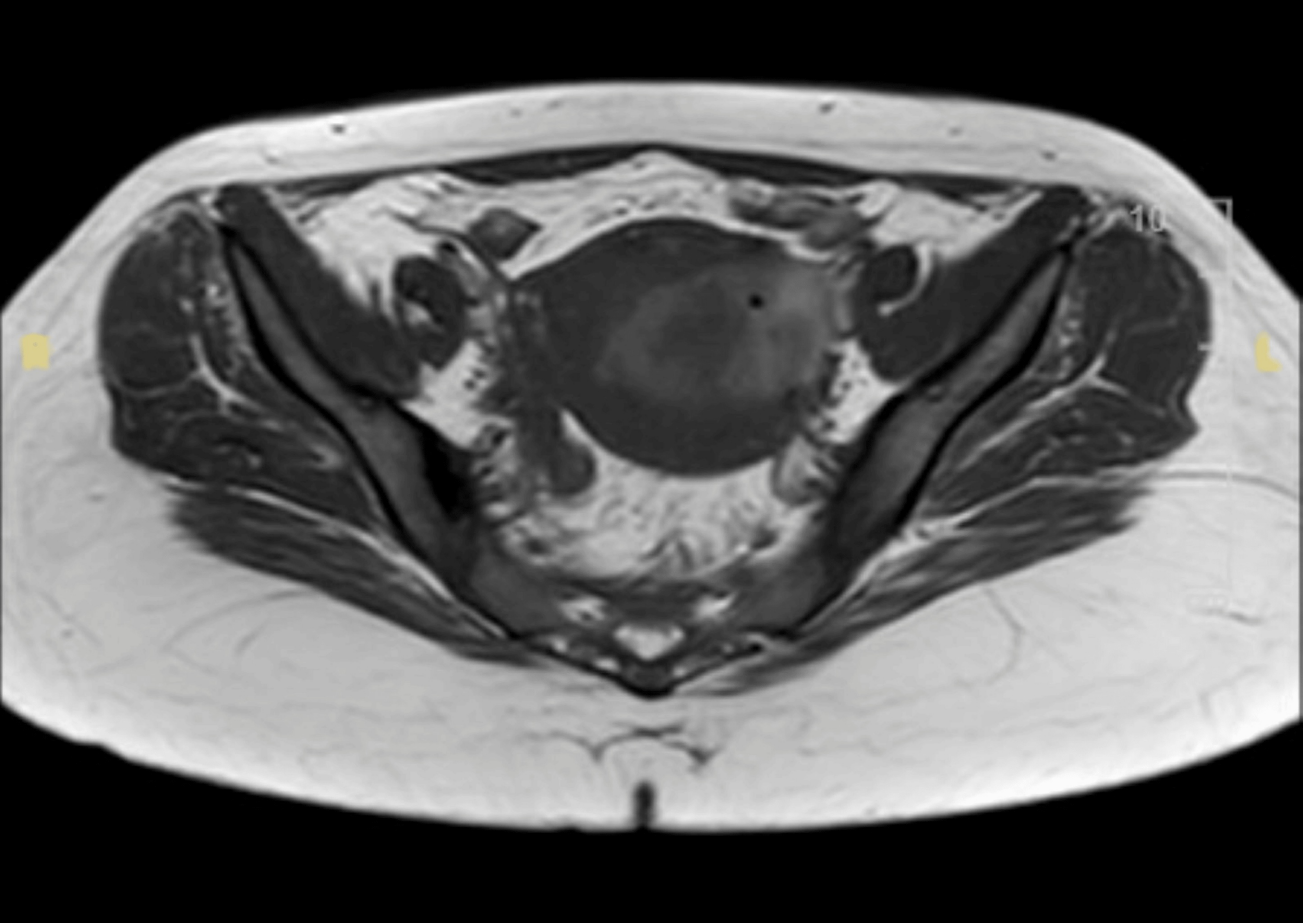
MRI reported as follows: appearances are suspicious of uterine rupture/dehiscence.
